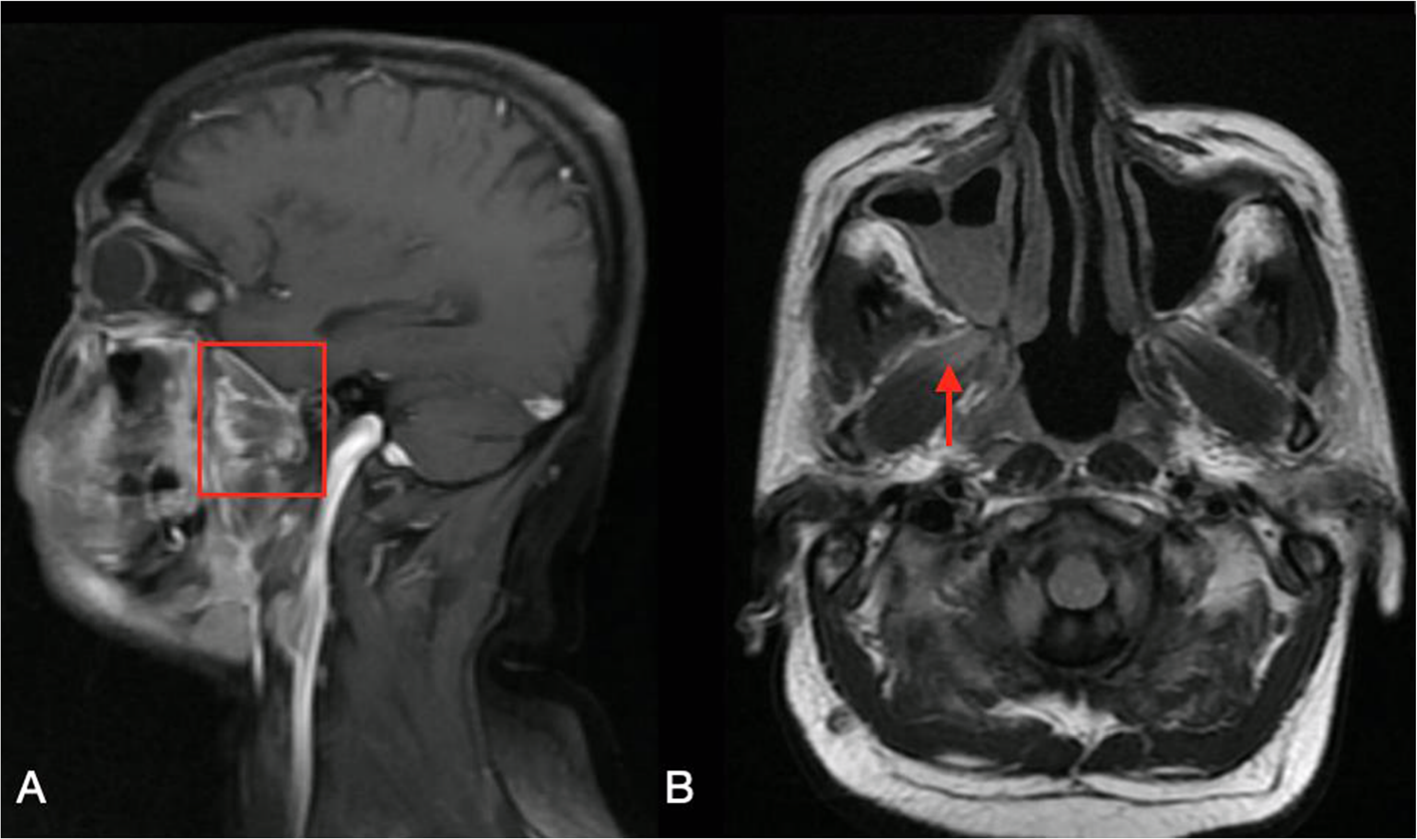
T1 sagittal FS + contrast (A) and axial FLAIR (B) study there is enhancement of right medial and lateral pterygoid muscles (red box) and subcutaneous soft tissue component anterior to maxilla on right side. Inflammation and involvement of the right pterygoid muscles (red arrow) and parapharyngeal space below it
